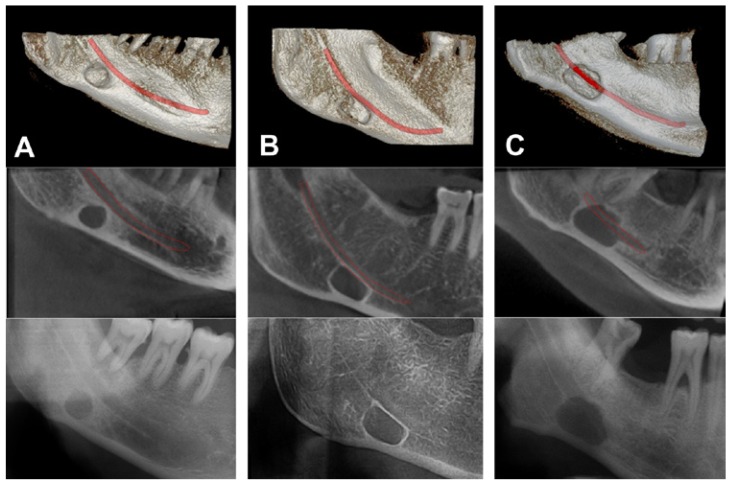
This figures show the SBCs relation to mandibular canal on CBCT images (3D and sagittal view) and panoramic radiographs. Three types of relation were observed.
